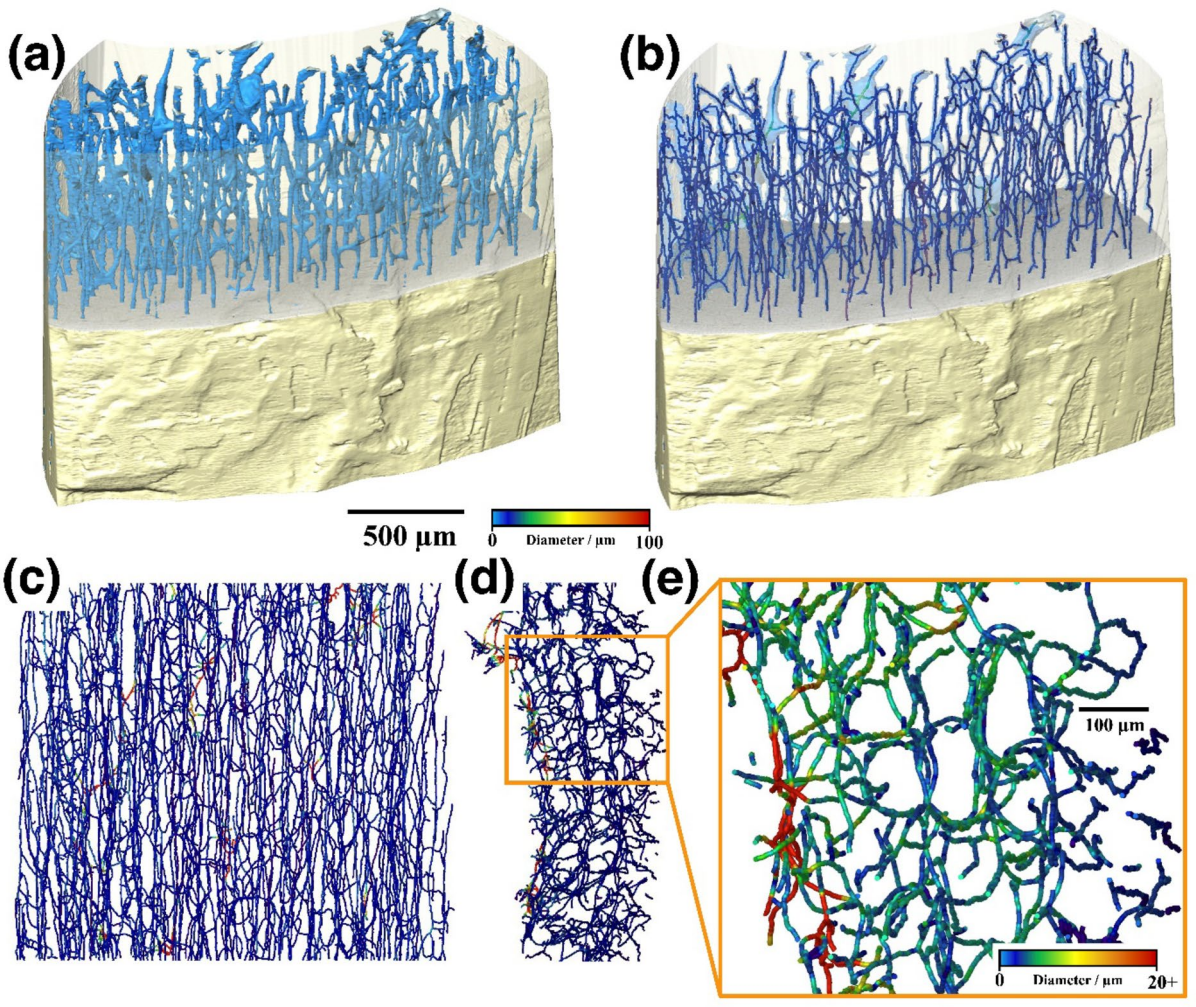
Rhamphorhynchus* sp. (OUMNH PAL-J.028331) rendering (a) and canal skeletonised network visualisation (b–d) after segmentation from cortical bone. (a) Shows a 3D rendering of the CT scans, with the top half semi-transparent, showing the canals’ (blue) distribution throughout the bone. (b) Is similar to (a), but with the canal volume semi-transparent and instead shows the skeleton’s place in the canals. (c) Shows the canal network parallel to the bone’s longitudinal axis, and (d) shows the network perpendicular to the longitudinal axis where the bone’s outer edge is to the right. While most of the structure is homogeneous, there are a few areas where the canals get very large (the red areas in (c, d)). These are the large pores that can be seen in the CT data, which appear to connect to the rest of the canals. (e) Shows a magnified section of (d) with a smaller colour bar. There appear to be 3 different areas of the canal network. The inner section is filled with large pores (like those seen in Fig. 2), and the middle, appears to form a cellular pattern. The outer edge appears to have fewer canals, though this could be a consequence of some beam hardening.
